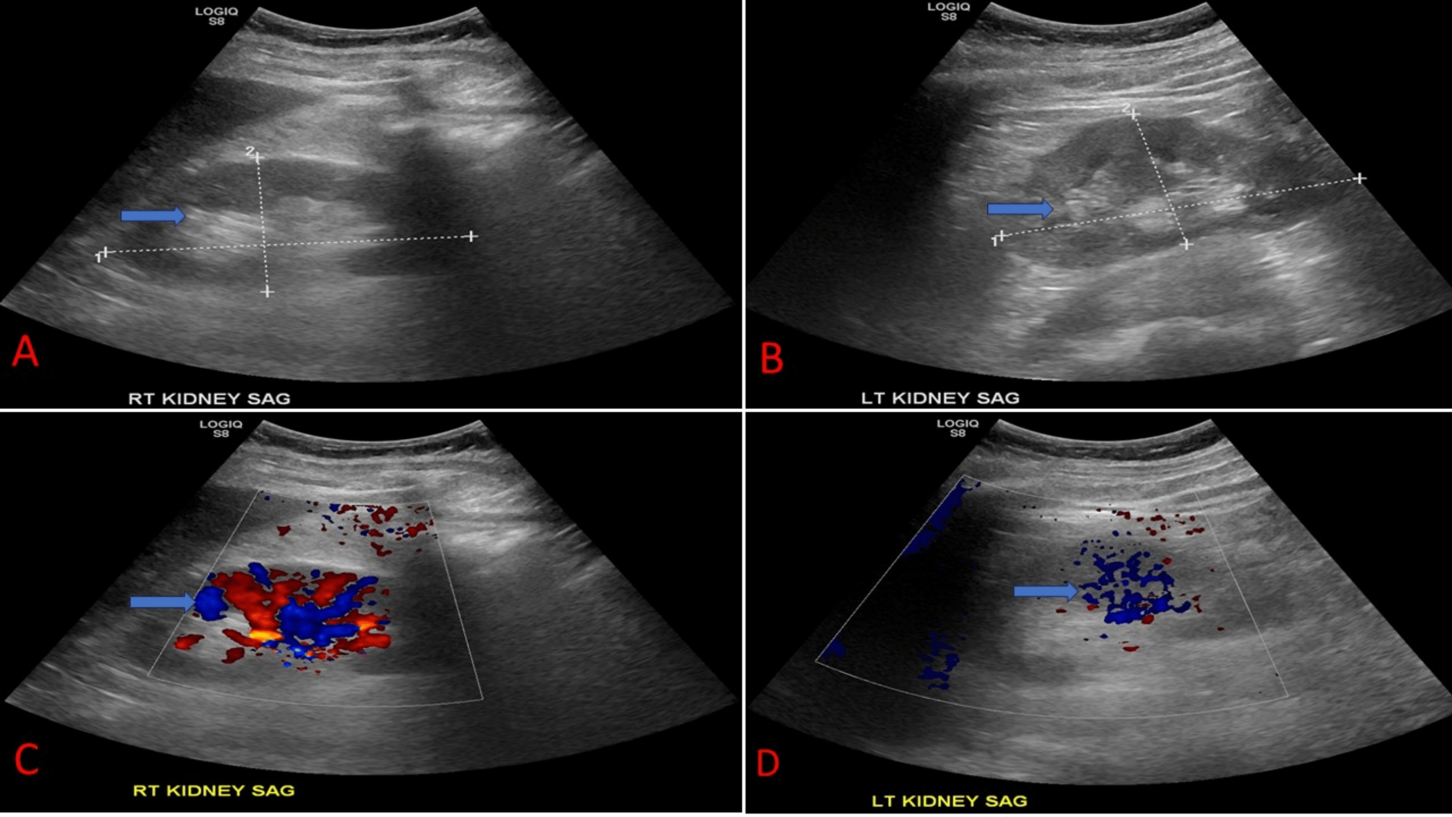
Kidney ultrasound of our patient.(A) Right kidney of size 10.6 x 5.3 x 5.0 cm with increased corticomedullary differentiation (blue arrow). (B) Left kidney of size 10.6 x 5.3 x 4.9 cm with increased corticomedullary differentiation (blue arrow). (C) Doppler images showing blood flow (blue arrow) of the right kidney. (D) Doppler images showing blood flow (blue arrow) of the left kidney
